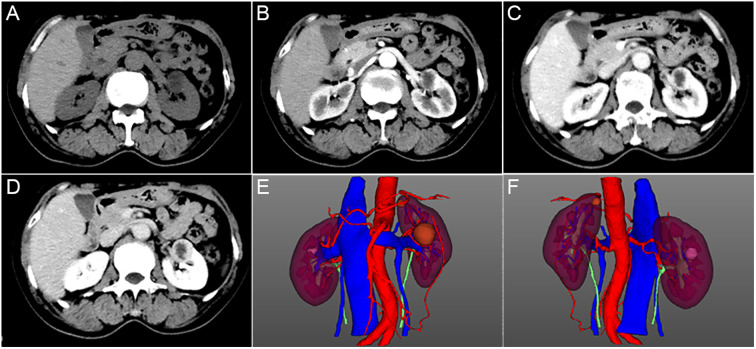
CT imaging and three-dimensional reconstruction. (A–D) Enhanced CT of the lower abdomen showing a round-shaped, slightly low-density cyst measuring approximately 24×28 mm in size in the left kidney. The lesion shows peripheral rim enhancement on contrast-enhanced scans. (E, F) Three-dimensional images of anterior view and dorsal view are reconstructed.
